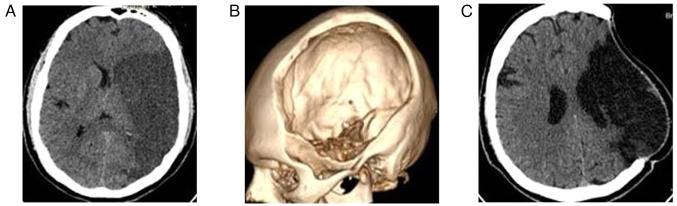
Fronto-temporoparietal craniectomy in a patient with malignant middle cerebral artery stroke (male patient, 48 years of age, with ischemic stroke into the left middle cerebral artery who underwent a left-sides craniectomy and right hemiplegia). (A) Pre-operative CT scan, axial view; (B) 3D craniectomy; (C) postoperative CT scan, axial view.
